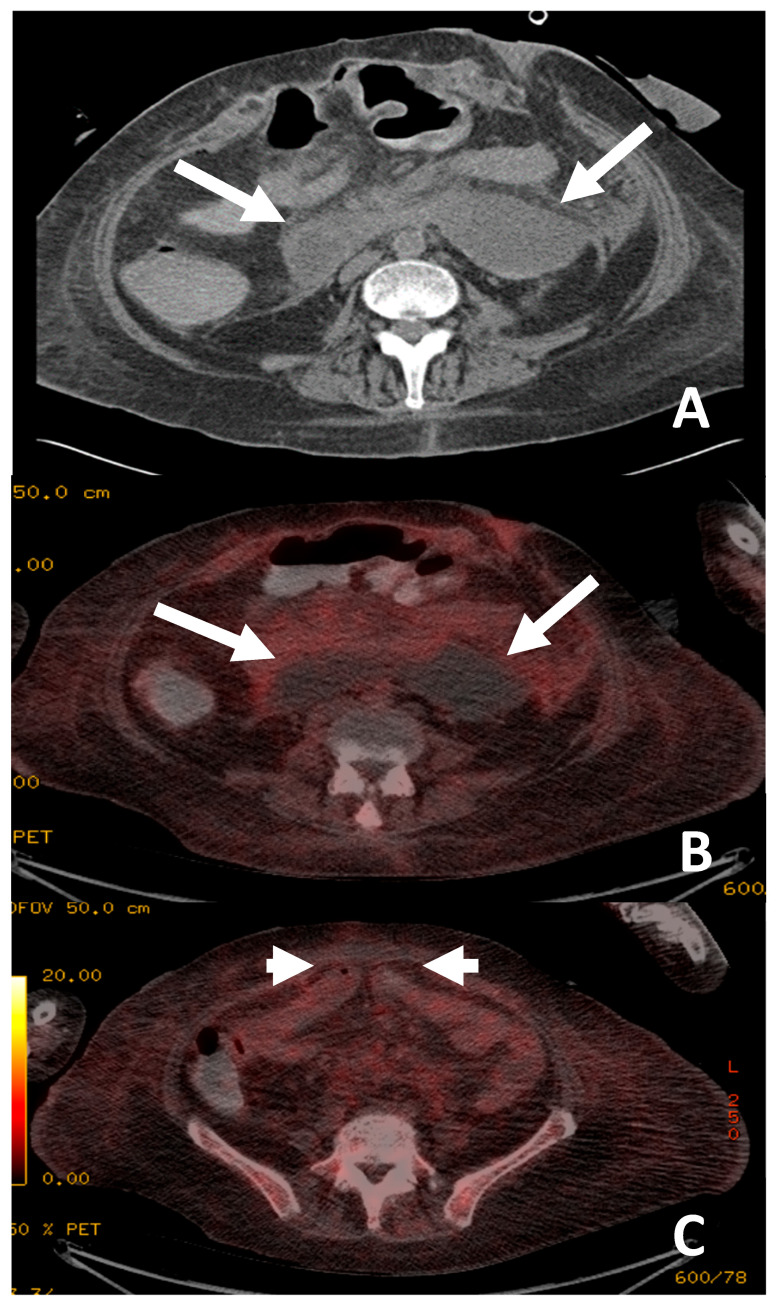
The patient presented with continued pyrexia and a white cell count of 40 × 10^9^cells/L. CT from 4 days prior demonstrated a lesser sac collection (A) with clinical concerns that the lesser sac collection (white arrows, image (A)) may be the source of sepsis and the permacol gusset mesh used for closure may be infected. ^18^F-FDG PET/CT (B,C) demonstrated the collection in the lesser sac (white arrows, image (B)) is likely to be innocuous as it did not show high tracer uptake and there was no significant uptake at the site of the permacol gusset closure material (short arrows, image (C)) to suggest an infected implant. The patient avoided a repeat operation for a surgical washout of the lesser sac collection and the removal of the closure mesh.
